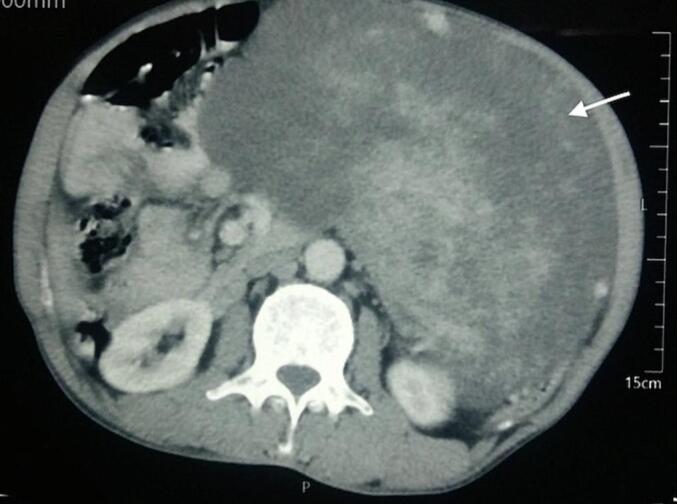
Contrast-enhanced CT abdomen showing a large, well-defined, mixed density tumor occupying the left side of the abdomen without distinct origin (arrow).
